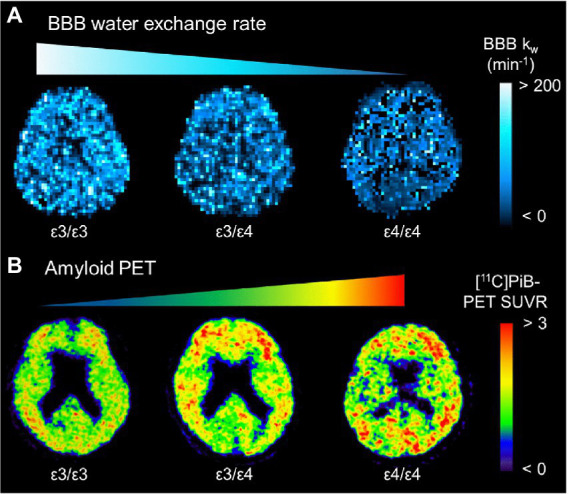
Representative BBB *k_w_* map (A) and [^11^C]PiB-PET SUVR (B) from an *APOE ɛ4* noncarrier (*ε3/ε3*), a heterozygote (*ε3/ε4*), and a homozygote (*ε4/ε4*). The *k_w_* map from the homozygote (*ε4/ε4*) displays the lowest *k_w_* values, which are associated with the highest SUVRs of [^11^C]PiB-PET. *APOE*: apolipoprotein E; BBB, blood–brain barrier; PiB, Pittsburgh compound B; SUVR, standard uptake value ratio [adapted with permission from Uchida et al. (2022a)].
